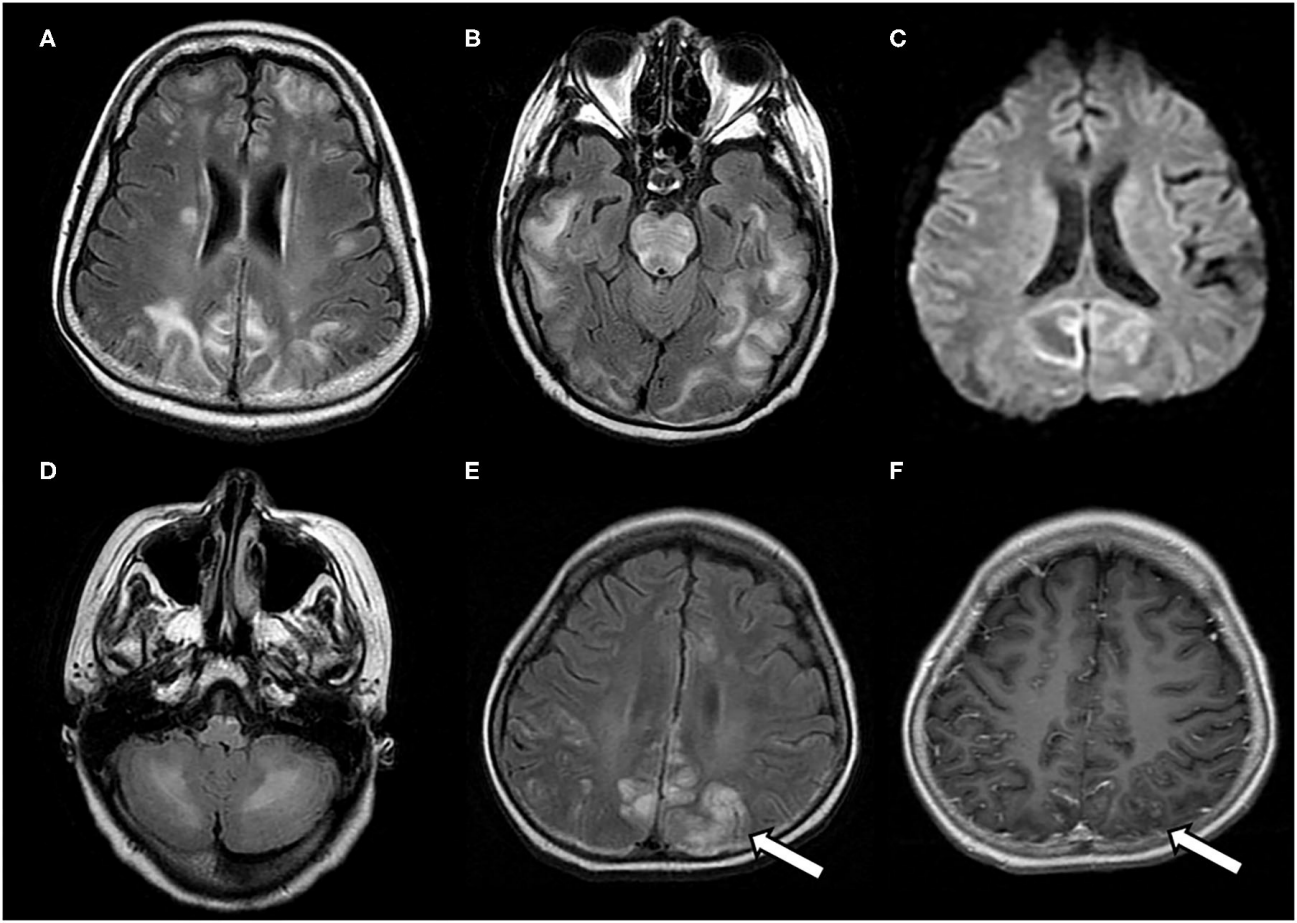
Atypical neuroimaging findings of PRES. (A,B) In a 17-year-old female with underlying systemic lupus erythematosus, lesions diffusely involving cerebral hemispheres, basal ganglia, and brain stem, with a prominent asymmetry on FLAIR images. (C) In a 12-year-old female with nephritic syndrome, MRI showed paradoxically hyperintense lesions on DWI (restricted diffusion) in bilateral occipital lobes. (D) In a 14-year-old male with membranoproliferative glomerulonephritis who showed prominent lesions of bilateral cerebellar hemispheres in FLAIR images (E,F) In an 8-year-old male with nephrotic syndrome, MRI showed occipital lesions hyperintensities on FLAIR, which also demonstrate post-contrast enhancement on T1-weighted image (white arrows).
